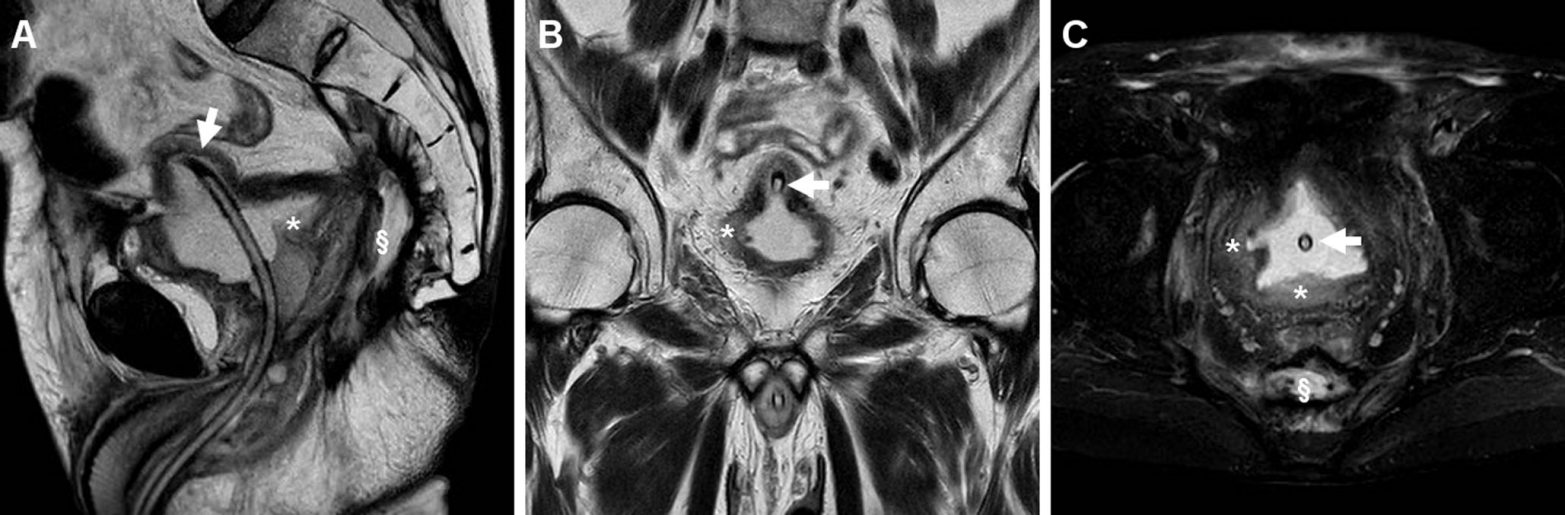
Acute infectious cystitis in 66-year-old bedridden man with several comorbidities and urosepsis. After inconclusive abdominopelvic CT (not shown), MRI showed contracted bladder with Foley catheter (*thick arrows*) and diffuse mural thickening with multifocal high-signal oedematous regions, best appreciated with fat saturation (c)
